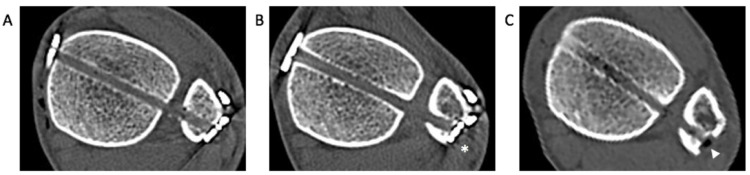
Sequential axial images of computed tomography showing cortical lysis of the fibula following suture button (SB) fixation: (A) immediate postoperative image showing intact fibular cortex facing the oblong button of the SB; (B) a year after initial fixation, medial translation of the oblong button is noticed (asterisk) with a mild widening of the tibiofibular space; (C) after SB removal, definite cortical lysis of the fibula is observed (white arrowhead).
